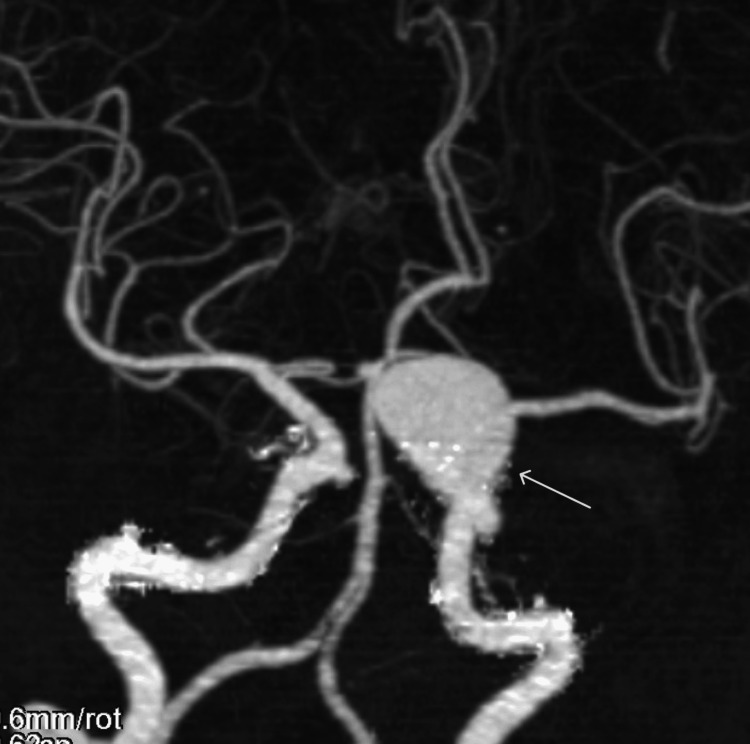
Coronal MIP CT angiographic image depicting a left ICA aneurysm (white arrow) MIP: maximum intensity projection, CT: computed tomography, ICA: internal carotid artery
